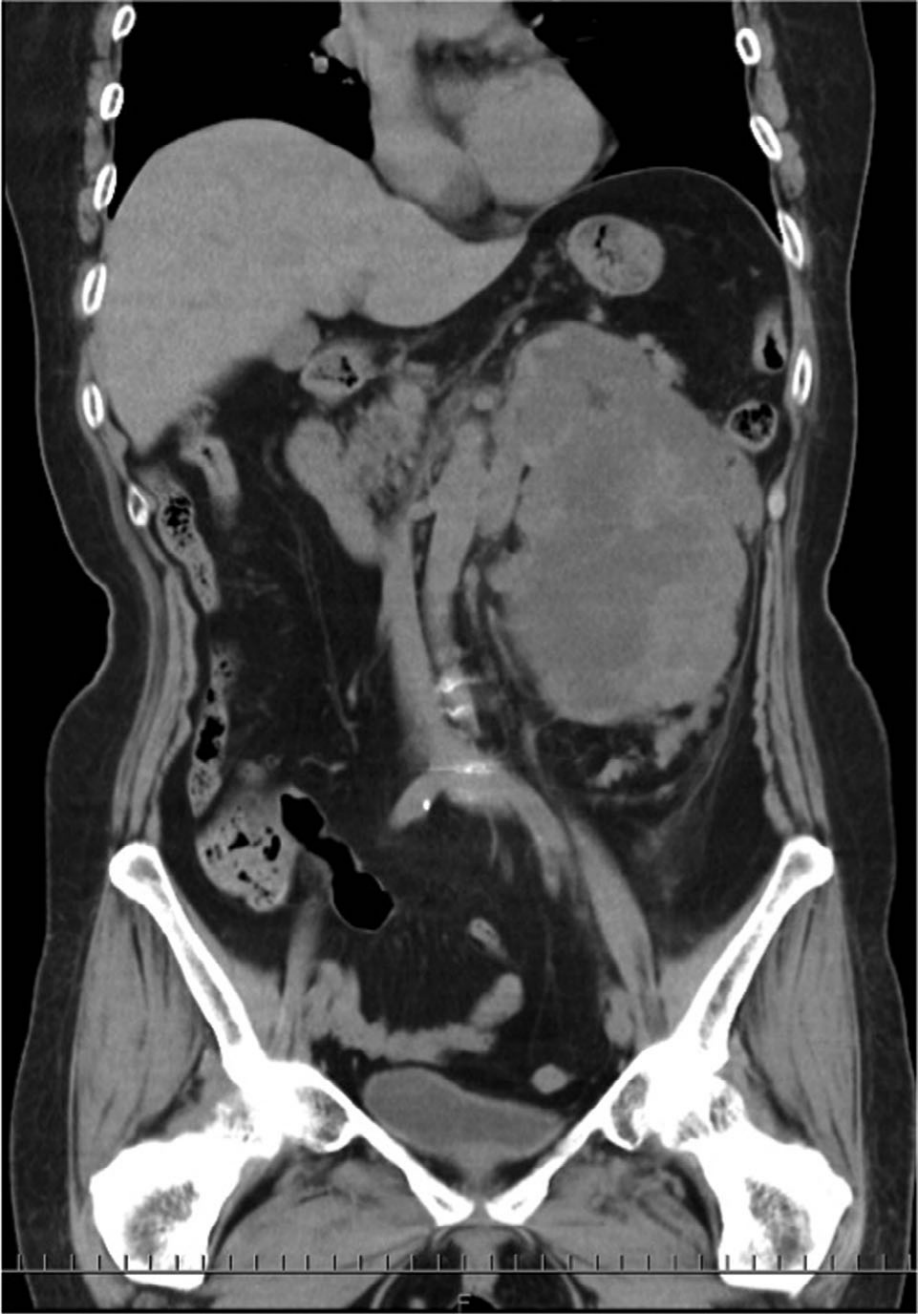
Computed tomography, coronal section showing left pelvic mass.
